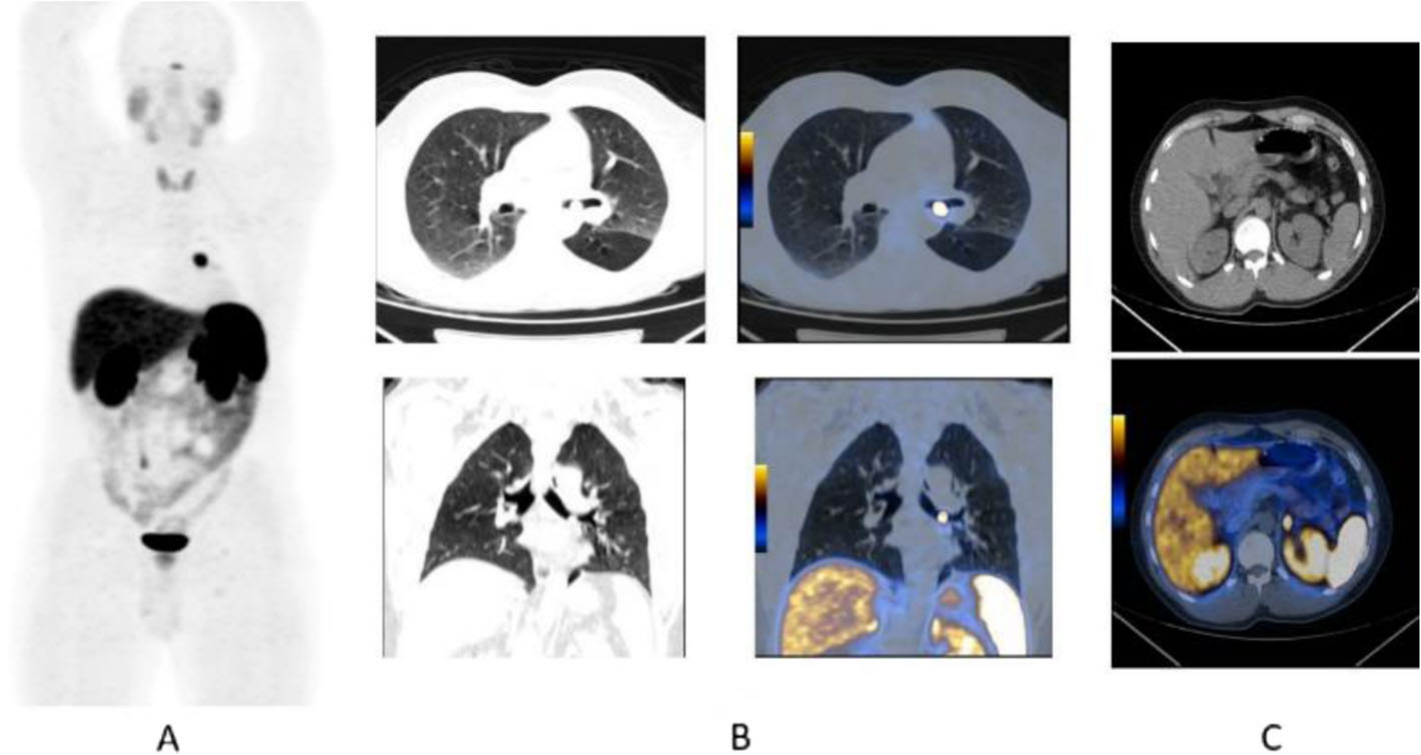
^68^Ga-DOTA peptide, staging of endobronchial carcinoid tumour, specificity. *Clinical history*: 33 y.o. man. Persistent cough and wheezing not responsive to bronchodilators. CT showed endobronchial node in left main bronchus. Bronchoscopy biopsy: NET. *PET/CT findings*: uptake in node protruding into the left main bronchus (a MIP, b CT and fused images). Focal uptake in the left adrenal gland (c). MRI confirmed the presence of an adrenal adenoma
